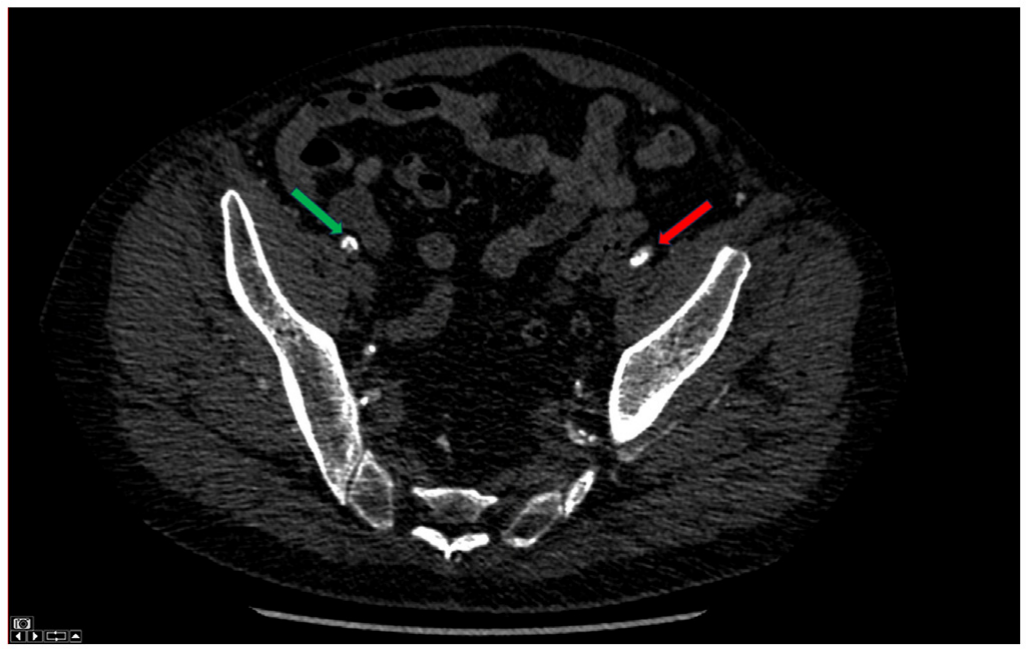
Computed tomographic angiography (CTA) reveals severe peripheral arterial disease of the right external iliac artery (EIA) (*green arrow*) and the left EIA (*red arrow*).
